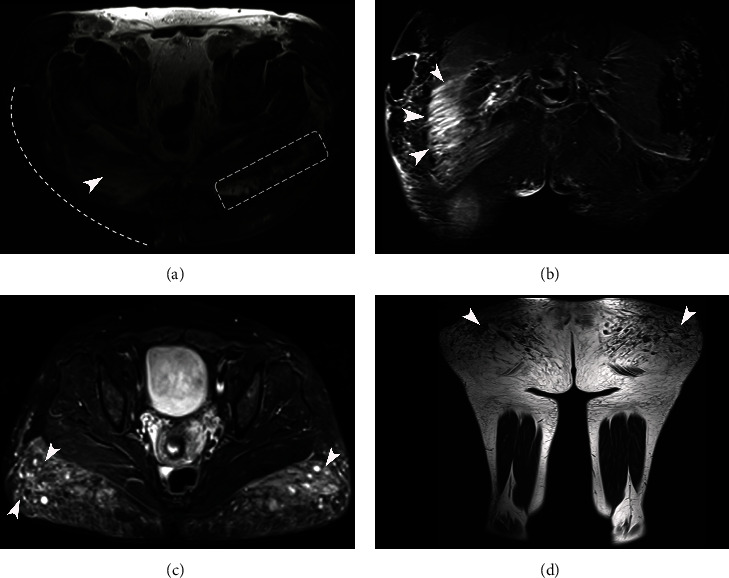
Magnetic resonance imaging STIR sequence applied in two different patients with complications in the gluteal region. Patient 1: (a) T2 axial sequence: the modeling material's presence affects all the subcutaneous cellular tissue of the hips with a diffuse pattern left gluteal region. Note the hypointense signal in this sequence, with inflammatory changes of the major gluteal muscles (dotted white rectangle); the patient presented resection of the subcutaneous cellular tissue of the right gluteal region due to a complication of necrosis (dotted white line) and can be observed the infiltration to the right gluteal muscle (white arrowhead). (b) Coronal sequence: the modeling material migration to the ischiorectal fossae (white arrowheads). Patient 2: unknown substance, an approximate amount of 1000 ml. (c) Axial plane shows infiltration with modeling material with a mixed globular and diffuse pattern that only affects the subcutaneous cellular tissue of the gluteal regions (white arrowheads). (d) Coronal plane: the signal of the modeling material is hypointense (white arrowheads).
